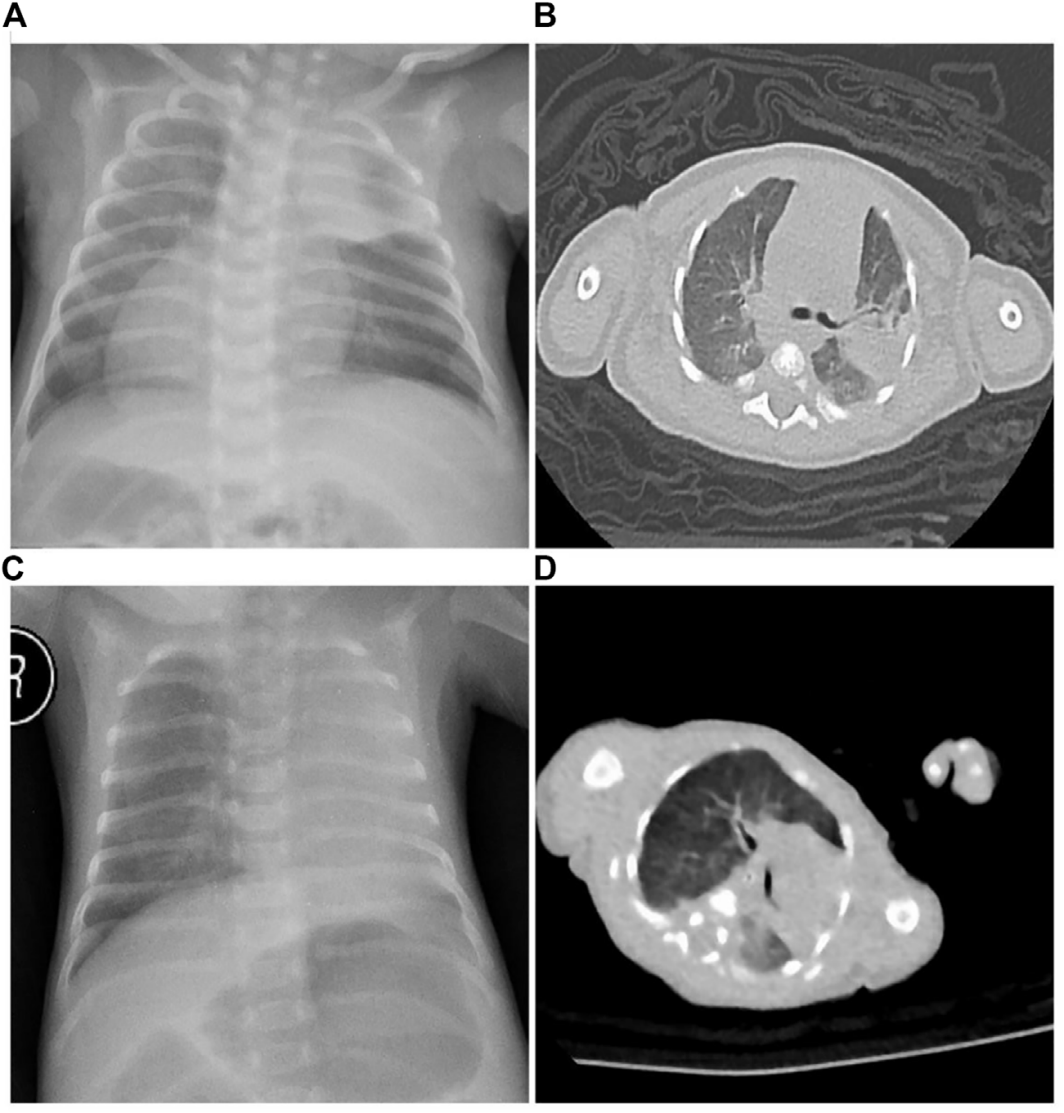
Radiological examination of the twins. (A) Twin A chest X-ray showed consolidation and atelectasis in the left upper lung, heart inversus and right-sided placement of the stomach bubble. (B) Twin A chest CT revealed bilateral lung infection with consolidation in the left upper lobe and mirror-image dextrocardia. (C) Twin B chest X-ray showed atelectasis in the left upper lung and normal cardiac and stomach placement. (D) Twin B chest CT revealed atelectasis in the left upper lung.
